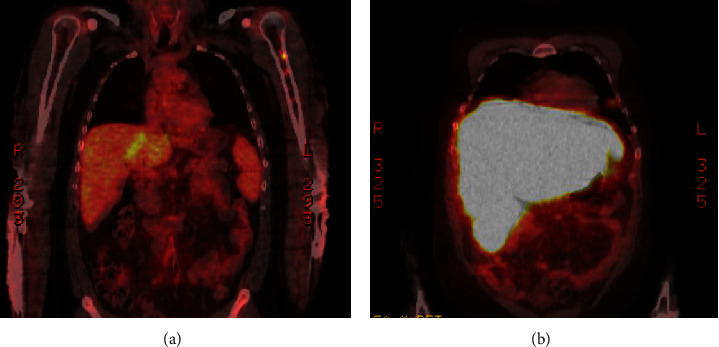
PET/CT scan of the abdomen. (a) One month prior to the first cycle of nivolumab: multiple intrahepatic lesions. (b) Day 22 after the first cycle of nivolumab: significant hepatomegaly due to hepatitis, significant increased metabolic activity of the entire liver, and new abdominal ascites. The PET/CT scan of the patient abdomen depicts the changes in the patient's liver before and after starting nivolumab. The second picture shows the large size of the liver when the patient developed autoimmune hepatitis.
